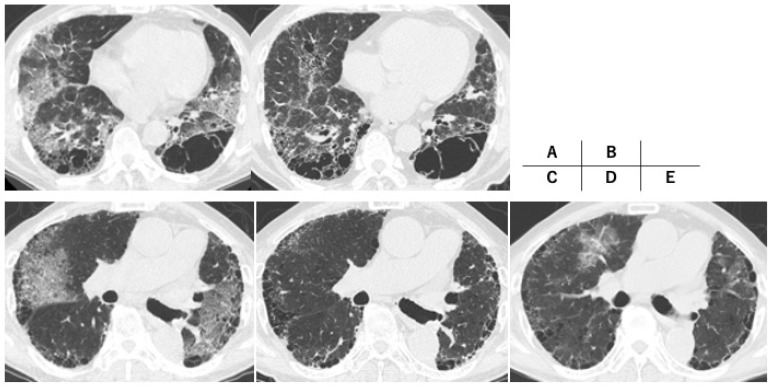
High-resolution computed tomography findings of severe cases. Case 1 (A,B): idiopathic pulmonary fibrosis (IPF), a 67-year-old man ((A): onset of COVID-19, (B): one year after onset of COVID-19); Case 2 (C–E): IPF, a 73-year-old man ((C): onset of COVID-19, (D): five months after onset of COVID-19, (E): acute exacerbation nine months after onset of COVID-19).
